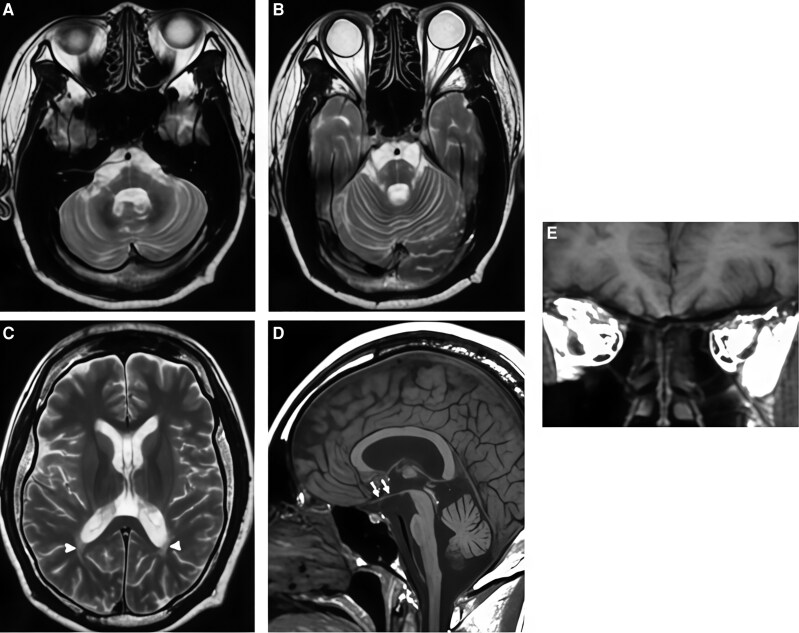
MRI brain showing optic nerve and cerebellar/brainstem atrophy in Wolfram syndrome; (A, B) Axial T2-weighted images at the level of the posterior fossa showing prominent cerebellar atrophy, with thinning of the cerebellar hemispheres and vermis (white arrows). (C) Axial T2-weighted image at the supratentorial level demonstrating mild ventricular prominence with diffuse cerebral volume loss, consistent with generalized cerebral atrophy (white arrowheads). (D) Mid-sagittal T1-weighted image showing marked cerebellar vermian and brainstem (pons and midbrain) atrophy, with thinning of the brainstem contour (white arrows). (E) Coronal image through the orbit demonstrating bilateral optic nerve atrophy, consistent with optic neuropathy (white arrows).
